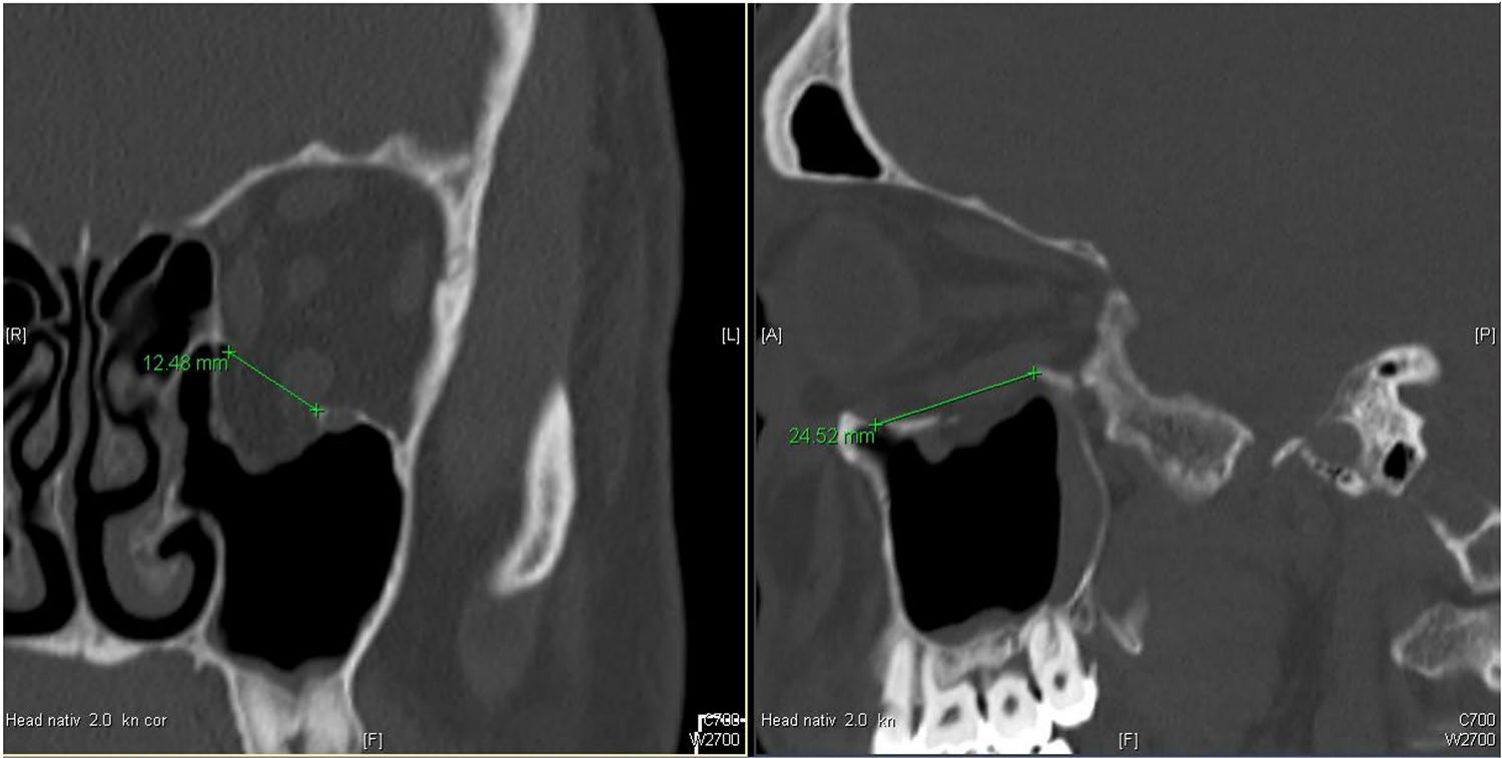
Coronal and sagittal CT slice of an isolated orbital floor fracture with a prolapse and incarceration of surrounding soft tissue. The size of the orbital floor defect was calculated by measuring the widest range of the defect in the coronal slices of the CT scans multiplied with the number of slices in which the defect was still visible and the layer thickness of the coronal slices
